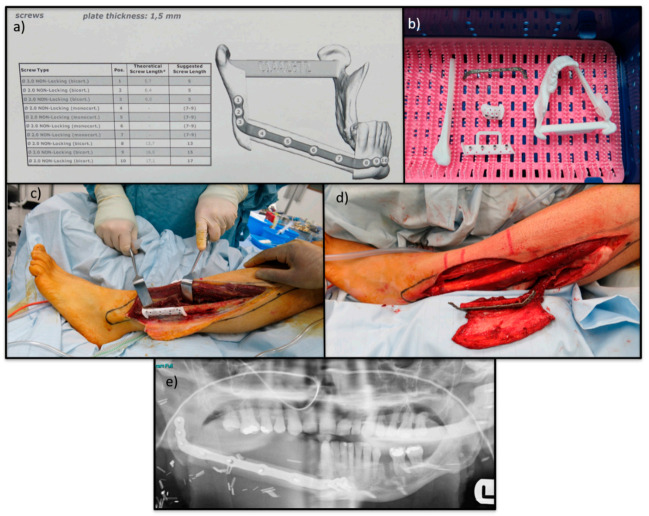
Images show the reconstruction of a mandibular OS. (a) Shows the reconstruction plan including the pre-determined screw lengths and locations. (b) Shows the 3D cutting guides, 3D model and the custom reconstruction plate. (c) Shows custom cutting guide on free fibula osseocutaneous flap. (d) custom reconstruction plate attached to free fibular osseocutaneous flap. (e) Shows the follow up orthopantomogram with free fibula osseocutaneous flap fixed in defect.
